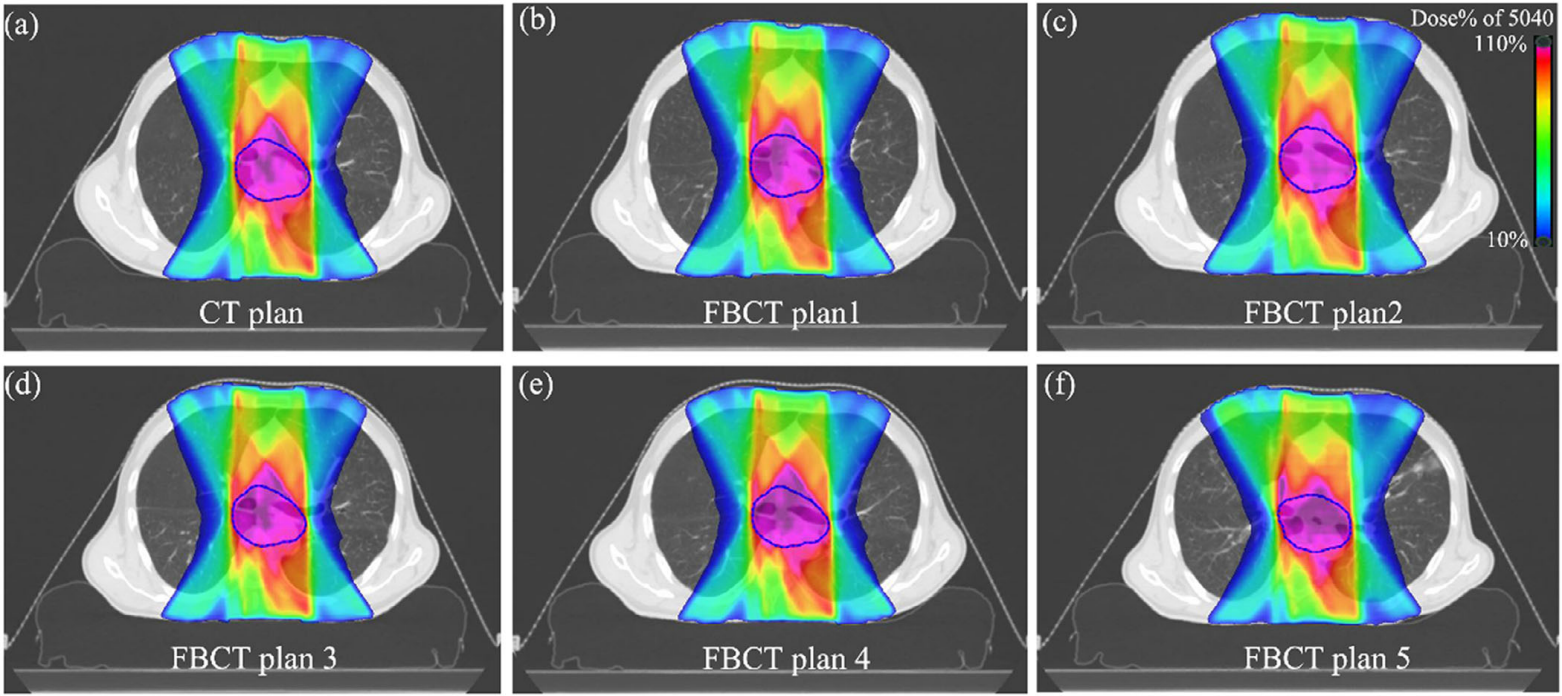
Dose distribution in a cross‐sectional view for a lung cancer patient (prescription: 5040 cGy) on (a) CT image and recalculated dose distribution with setup errors on FBCT; (b)‐(f) for different fractions.
